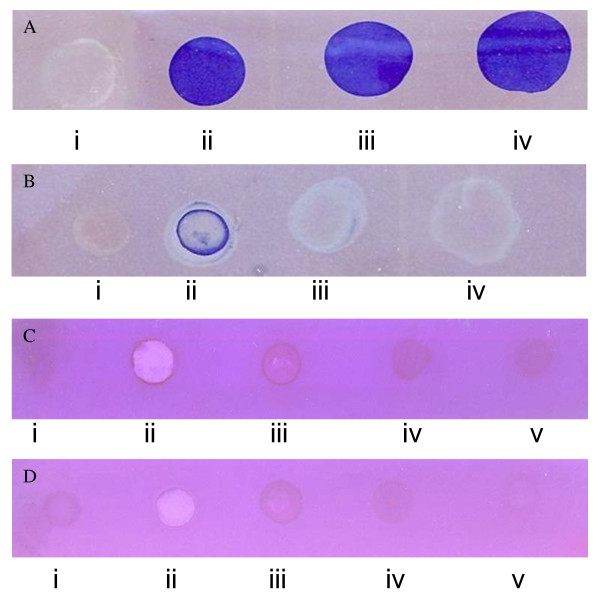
Detection of protease inhibitory activity using X-ray film. Trypsin (5, 10 & 15%, w/v in Tris-HCl buffer) cleared the gelatin coat on film (Figure 1A-ii, iii & iv). Buffer alone served as control (Fig. 1 A-i). Trypsin (10%), preincubated with heat-treated crude seed extract (2 μg ml^-1^) for 60 min., was moderately inhibited and partial gelatin clearance was observed (Figure 1 B-ii). At higher concentrations (5, 12.5 μg ml^-1^), trypsin was completely inhibited and no gelatin digestion was seen (Figure 1 B-iii, iv). The heat-treated plant material (no endoproteolytic activity) served as control (Fig1 B-i). The inhibitory effect against *Aspegillus flavus *and *Bacillus *sp. protease is shown in Figure 1 C & D respectively. *Aspegillus flavus *and *Bacillus *sp. protease caused gelatin digestion (Figure 1 C-ii & D-ii) which upon incubation with seed extract was not seen (Figure 1 C-iii, iv & D-iii, iv). Buffer alone (Figure 1C -i), heat treated (Figure 1D -i) and inactivated seed extract (Figure 1C -v & D -v) served as control.
